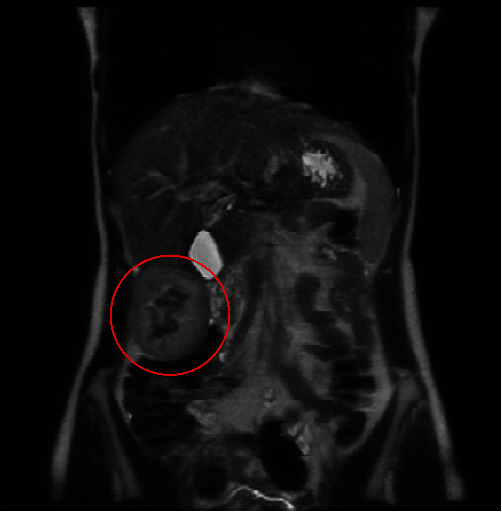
MRI showing nodular lesion arising from right hepatic lobe, in dynamic evaluation, after Gd–EOB–DTPA administration, heterogeneously captures the contrast product in a mainly peripheral way, and maintaining areas of central hyposignal.
